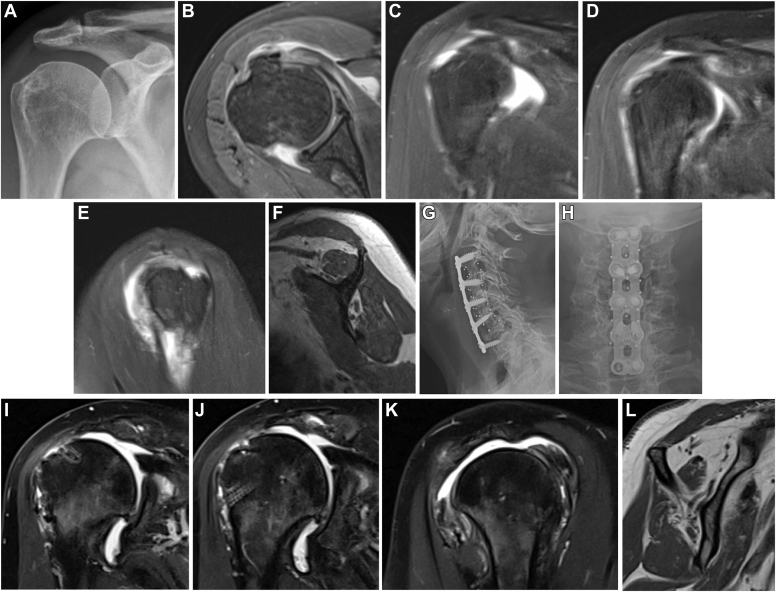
(A-E) Pre-operative images from clinical vignette #4. (G-H) Post-operative radiographic images from clinical vignette #4. (I-L) Post-operative MRI images from clinical vignette #4. *MRI*, magnetic resonance imaging.
